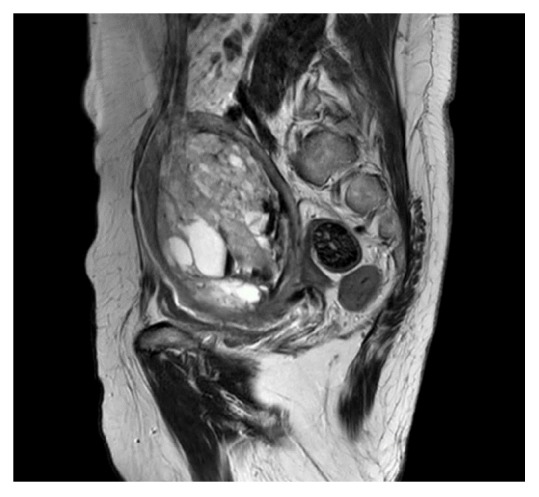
Large uterine tumor with deep myometrial invasion (MRI pelvis, T2 weighted sagittal).
